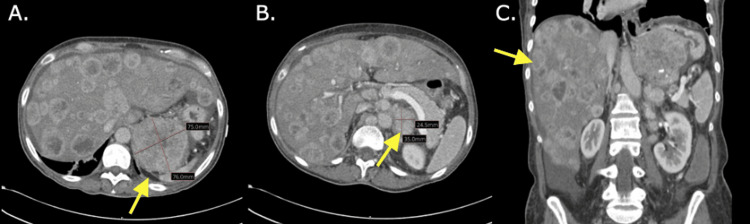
CT of the abdomen/pelvis with contrast. A: Transverse image of the CT showing a large 7.6 cm necrotic mass invading the proximal stomach. B: Transverse image of the CT showing multiple enlarged mesenteric, periaortic, and retroperitoneal lymph nodes, with the largest nodal mass in the left of the aorta measuring roughly 2.4 × 3.5 cm. C: Coronal image of the CT showing innumerable low attenuating liver lesions with peripheral enhancement consistent with diffuse metastatic disease.
